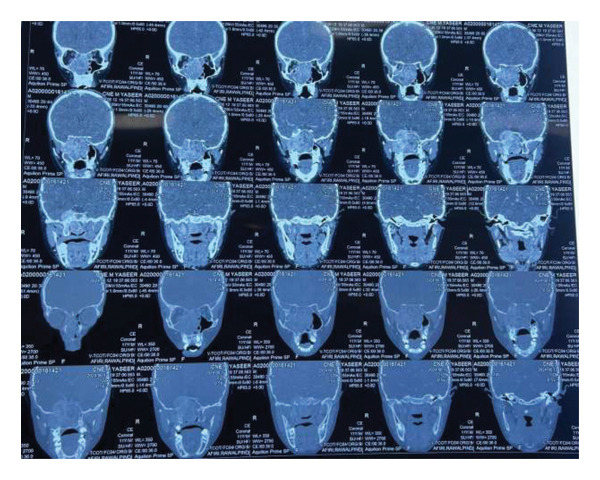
CT PNS with contrast coronal view: The lesion extends superiorly into the right pterygopalatine fossa, involving the right orbital apex and middle cranial fossa. There is encasement of adjacent neurovascular structures, with evidence of bony remodeling and destruction. The lesion extends posteriorly into the right sphenoid sinus and cavernous sinus, suggesting aggressive infiltration.
